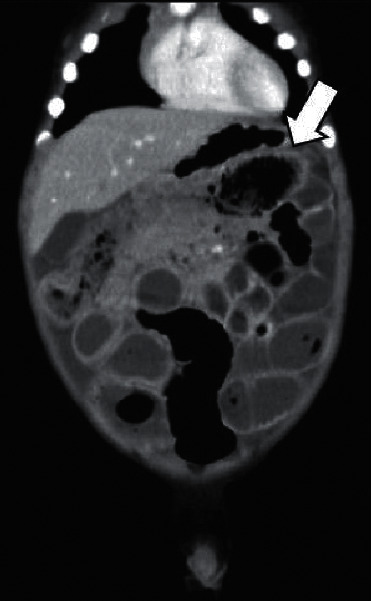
An abdominal computed tomography revealed fecalomas in the transverse colon (arrow), bowel distension from the jejunum to the transverse colon, and moderate ascites.
